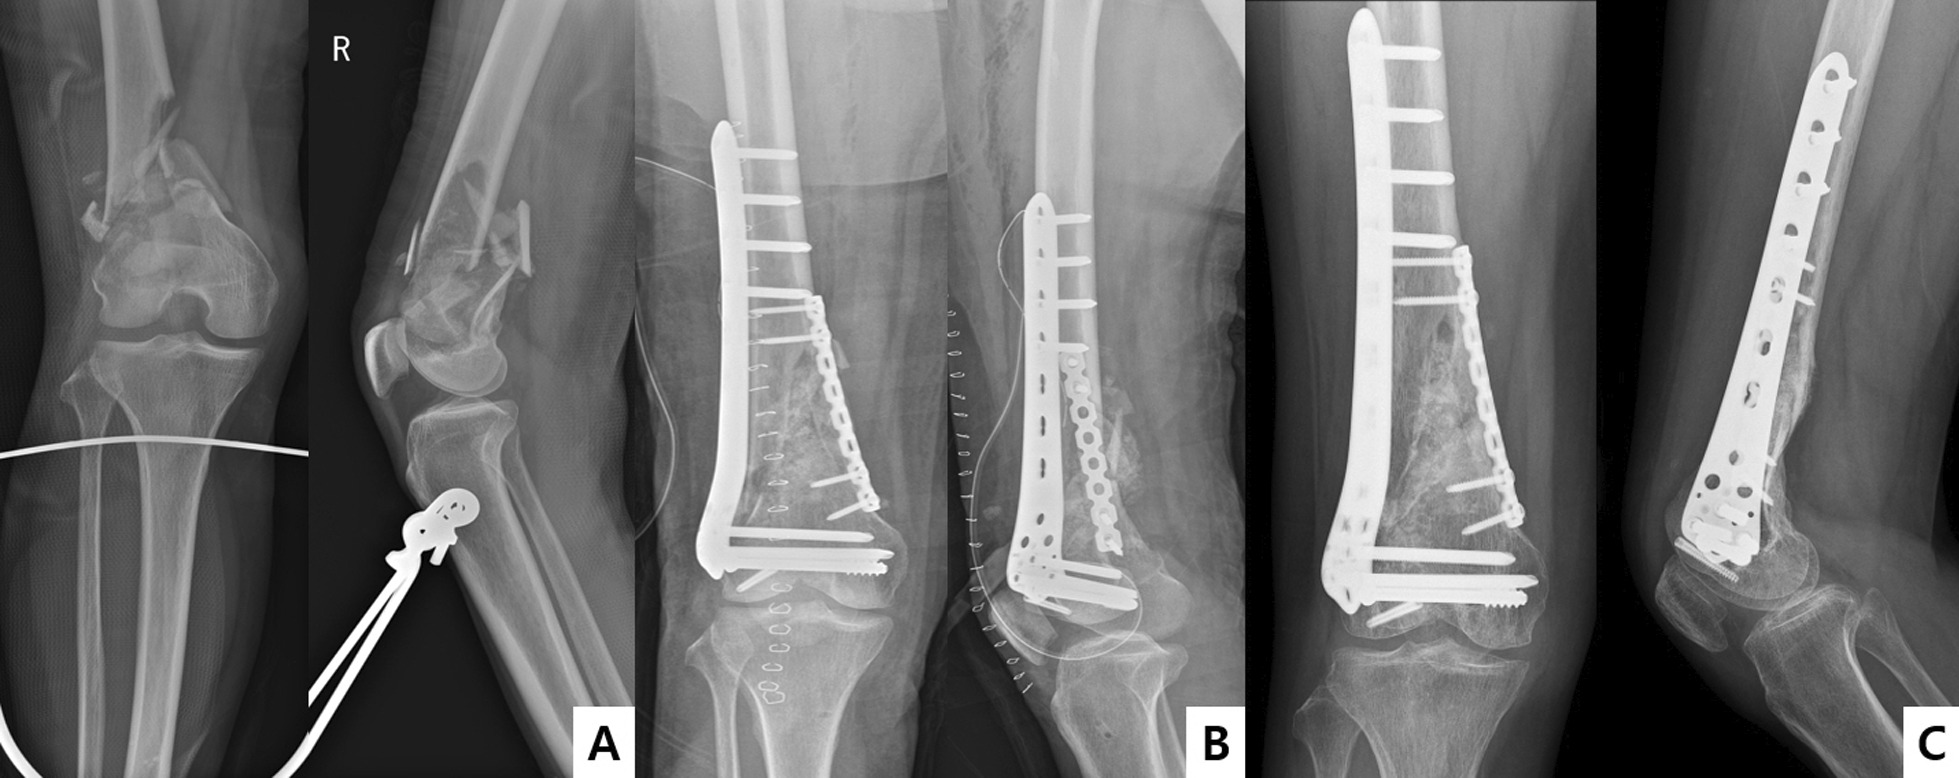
A Preoperative radiograph of a 70-year-old female patient showing a displaced distal femoral fracture. B Postoperative radiograph of the 70-year-old female patient showing the displaced distal femoral fracture. C Bone union was achieved at 6 months after the operation with double-plate fixation
